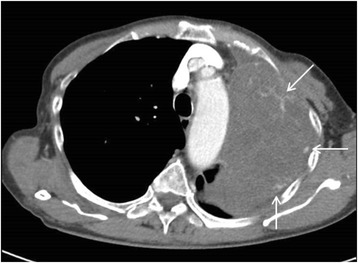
Computed tomography scan of the thorax revealed a large heterogenous mass in the left upper hemithorax with neovascularization (black arrows)
